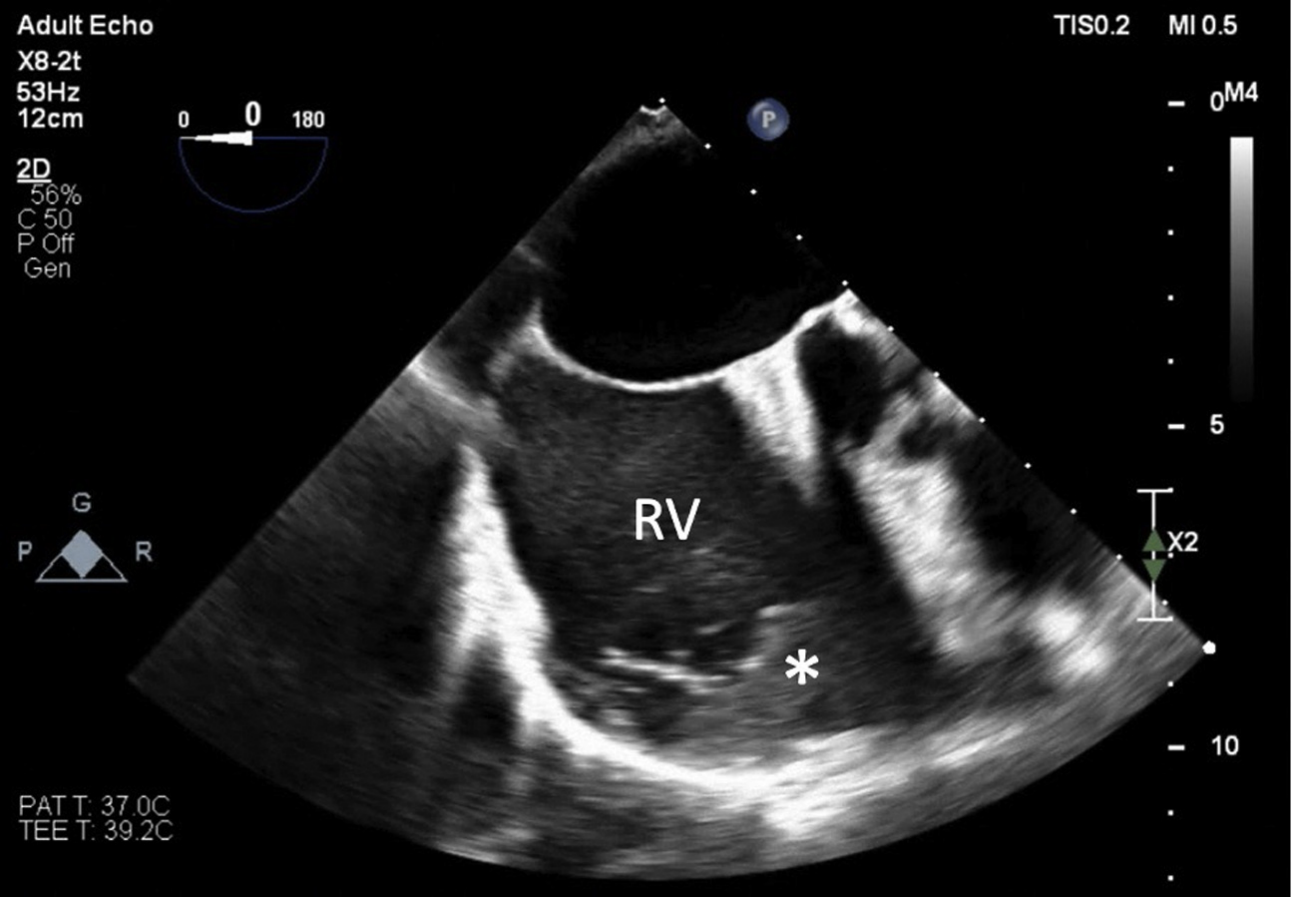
Echocardiographic Imaging Midesophageal echocardiographic view of the right atrium showing sludge or early thrombus (asterisk) forming in the right ventricle (RV) after transcatheter tricuspid valve repair.
